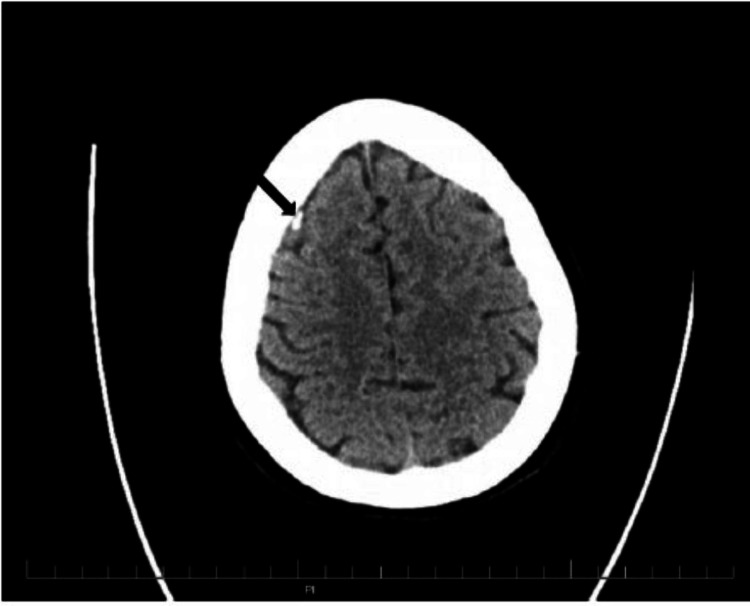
Head CT showing a 10 × 5 mm dense extra-axial calcification indicated with a black arrow.
